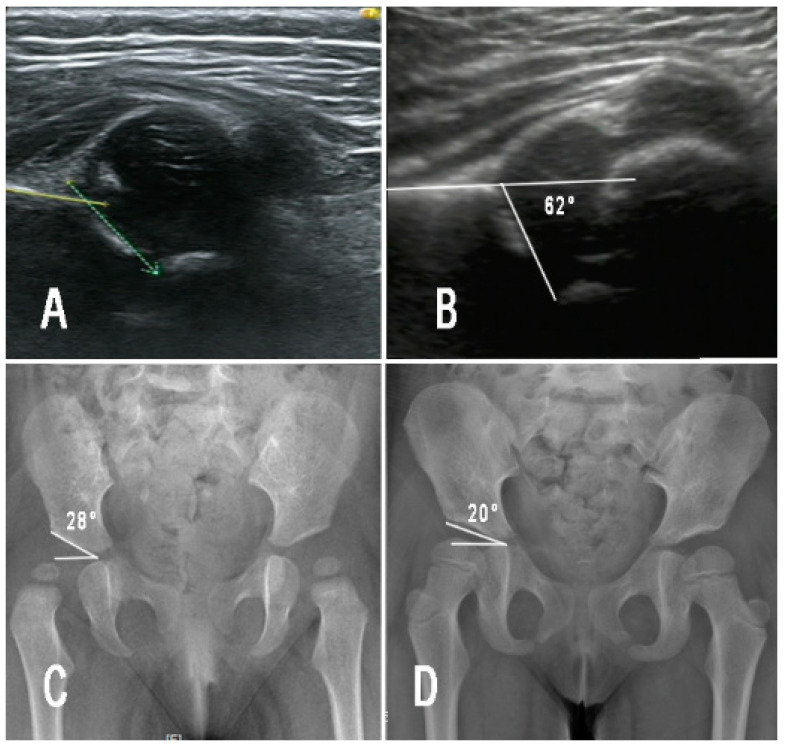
Female patient aged 3 months with right DDH (Graf type IV) (A), treated by PH for three months and achieved Graf type I (B), then the PH treatment was terminated. However, radiographic examination showed significant subluxation on the right hip 6 months later (C), and then the patient was treated by a night time abduction brace. At final follow-up (4 years old), the hip recovered to normal (D).
